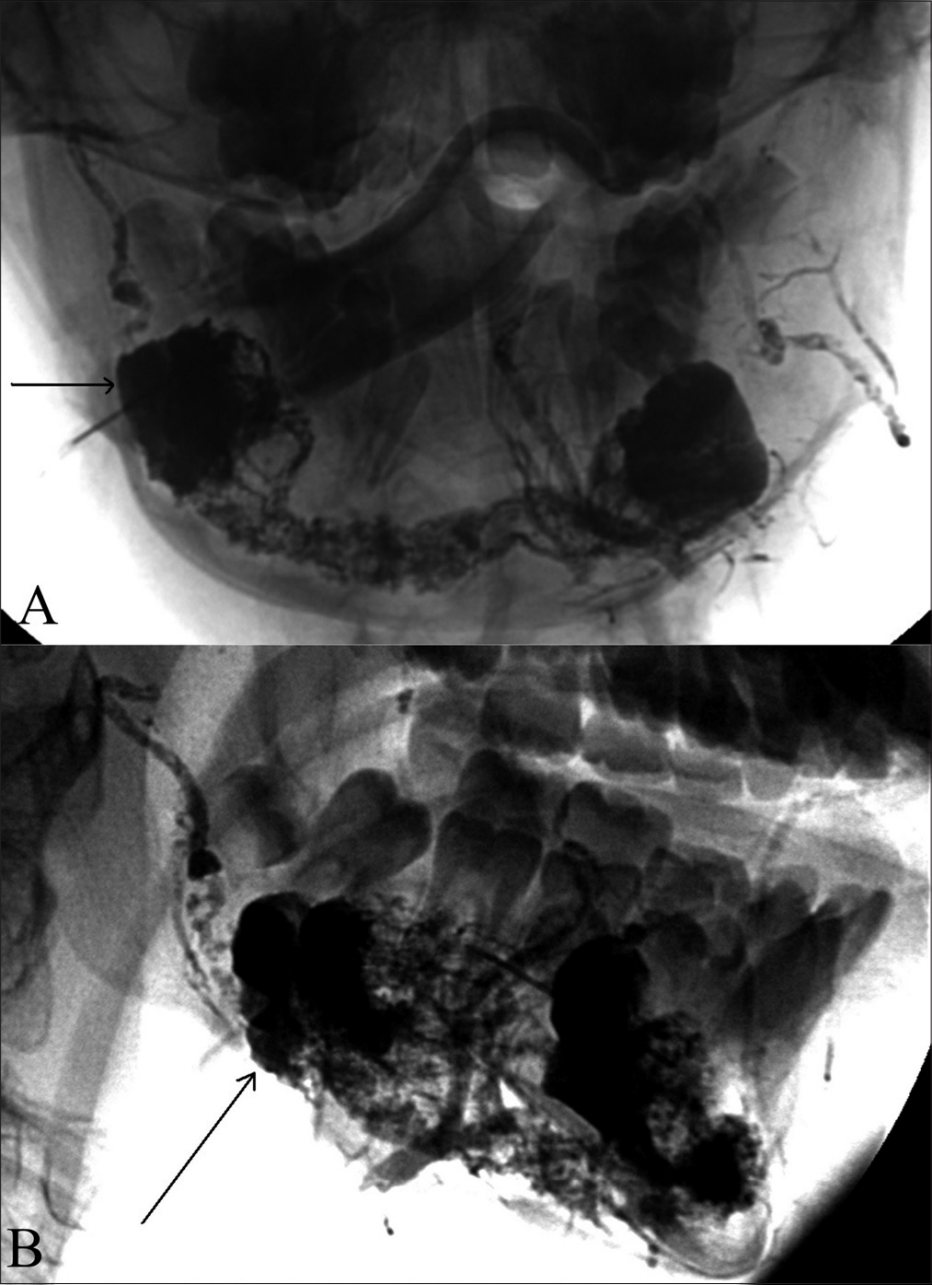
Frontal (A) and lateral (B) post-embolization radiographs after direct percutaneous glue injection show complete filling of the AVM (arrow) in the mandible with a mixture of N-butyl cyanoacrylate glue and radioopaque contrast agent (Lipiodol)
